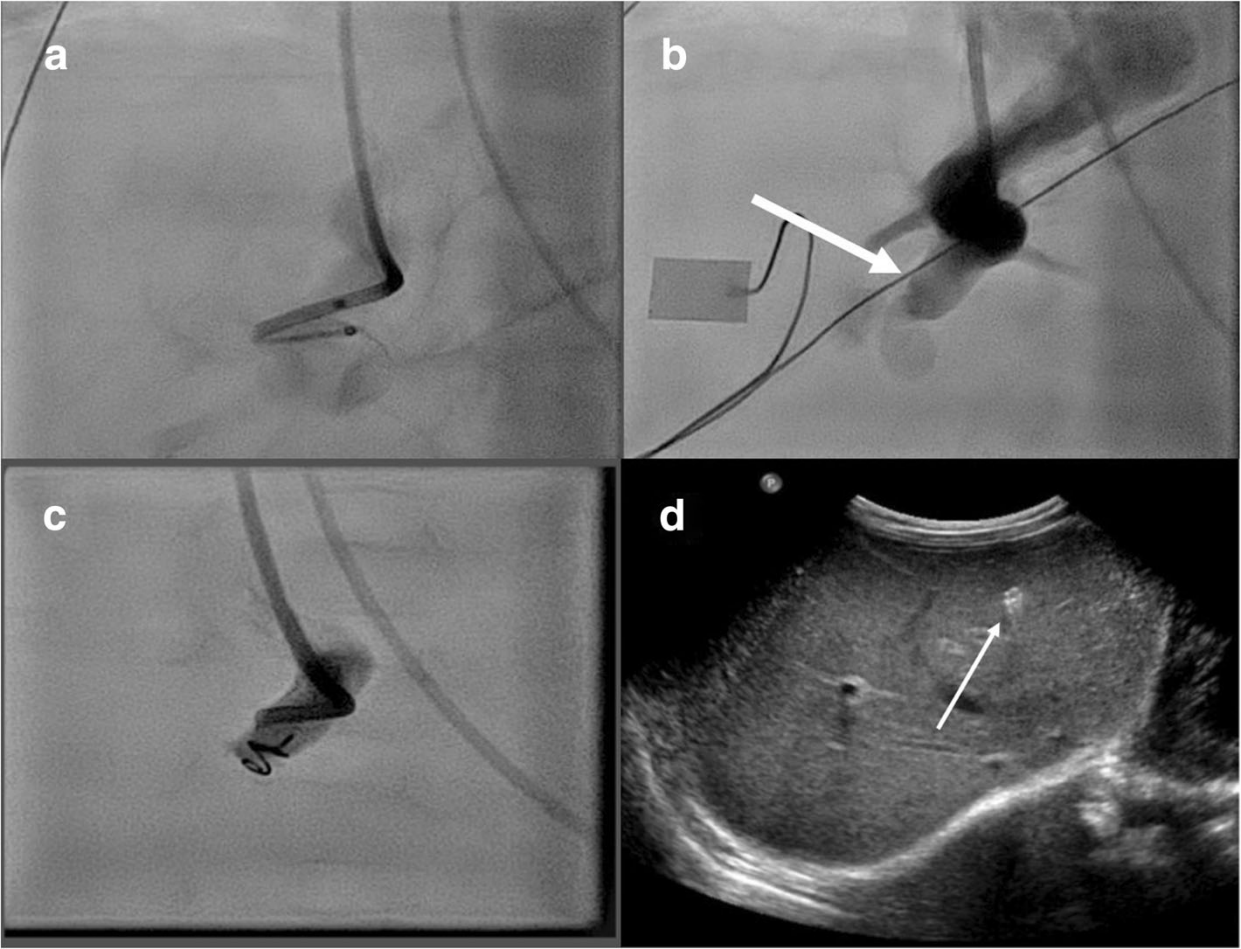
Closure of the remaining shunt. a–b The shunt (*white arrow*) during the intervention. c After the coil has been positioned. d Post-interventional control via ultrasound (coil marked by *white arrow*)
